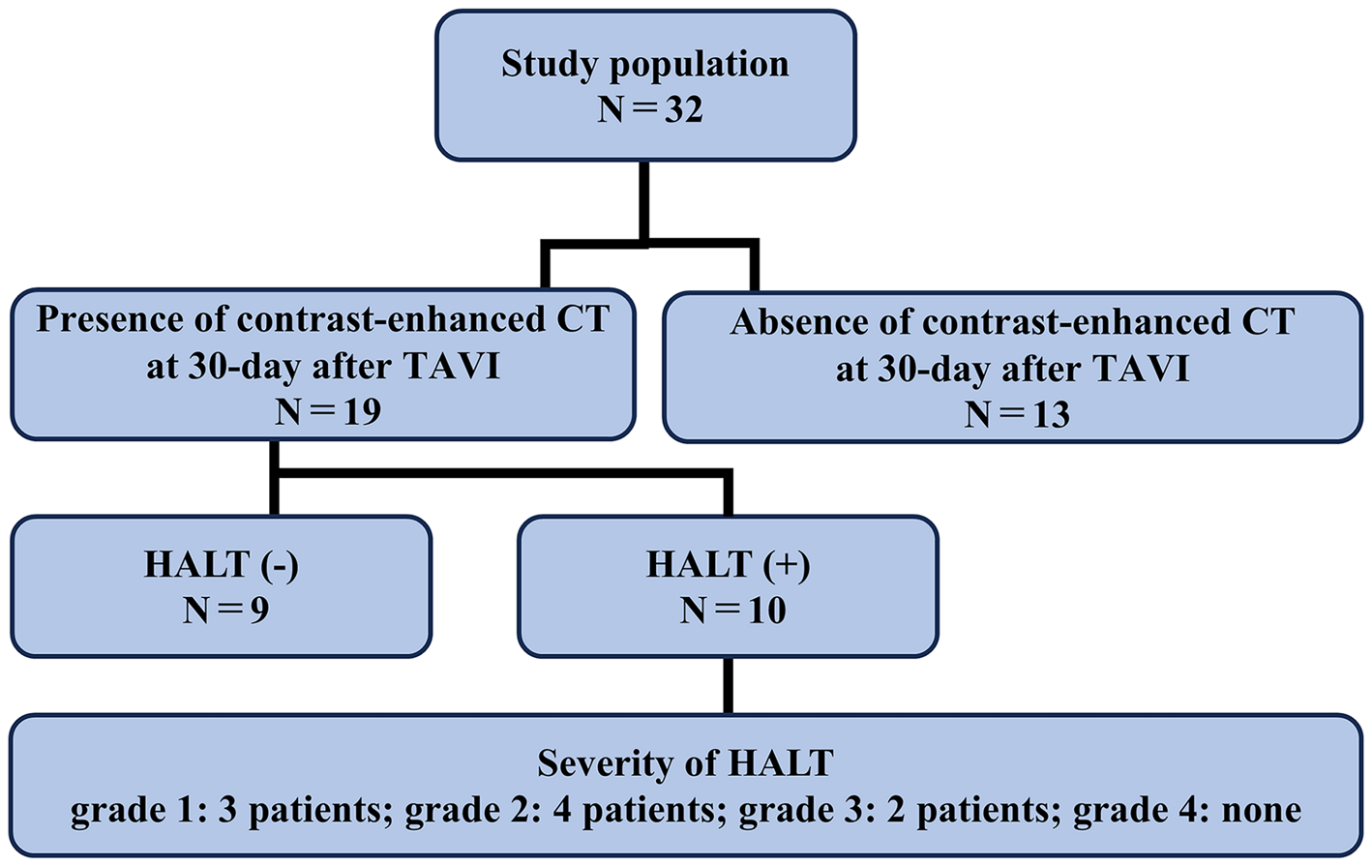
The presence and degree of HALT on contrast-enhanced CT 30 days after the procedure. Contrast-enhanced CT was performed 30 days after TAVI on 19 of 32 cases, with HALT observed in 10 cases. The severity of HALT was graded as follows: grade 1 in three, grade 2 in four, and grade 3 in three cases. *HALT* hypo-attenuated leaflet thickening, *TAVI* transcatheter aortic valve implantation, *CT* computed tomography
